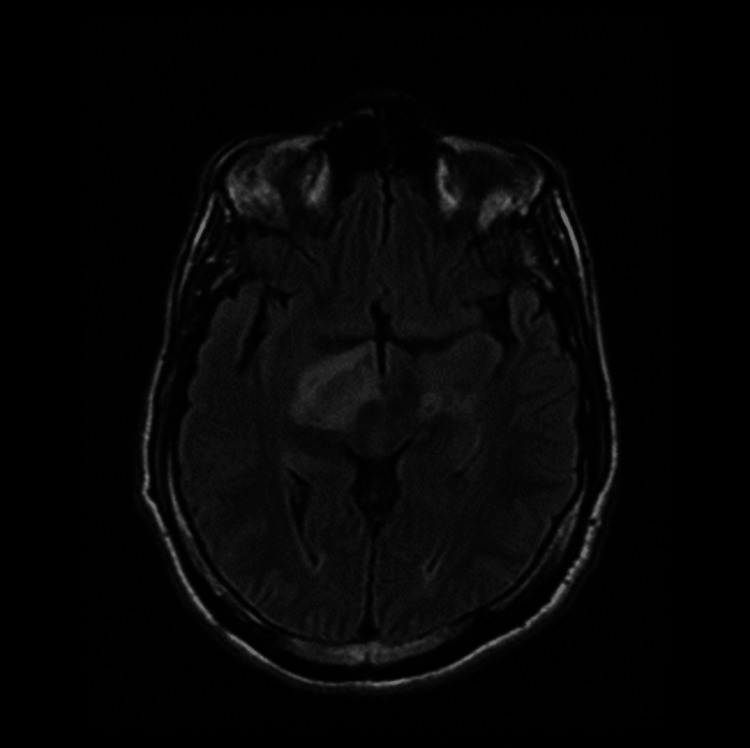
Brain MRI revealed abnormal signal intensity lesion seen at the right thalamus and right superior cerebellar peduncle reflecting hyperintense signals at FLAIR WI. FLAIR WI: fluid-attenuated inversion recovery-weighted image
